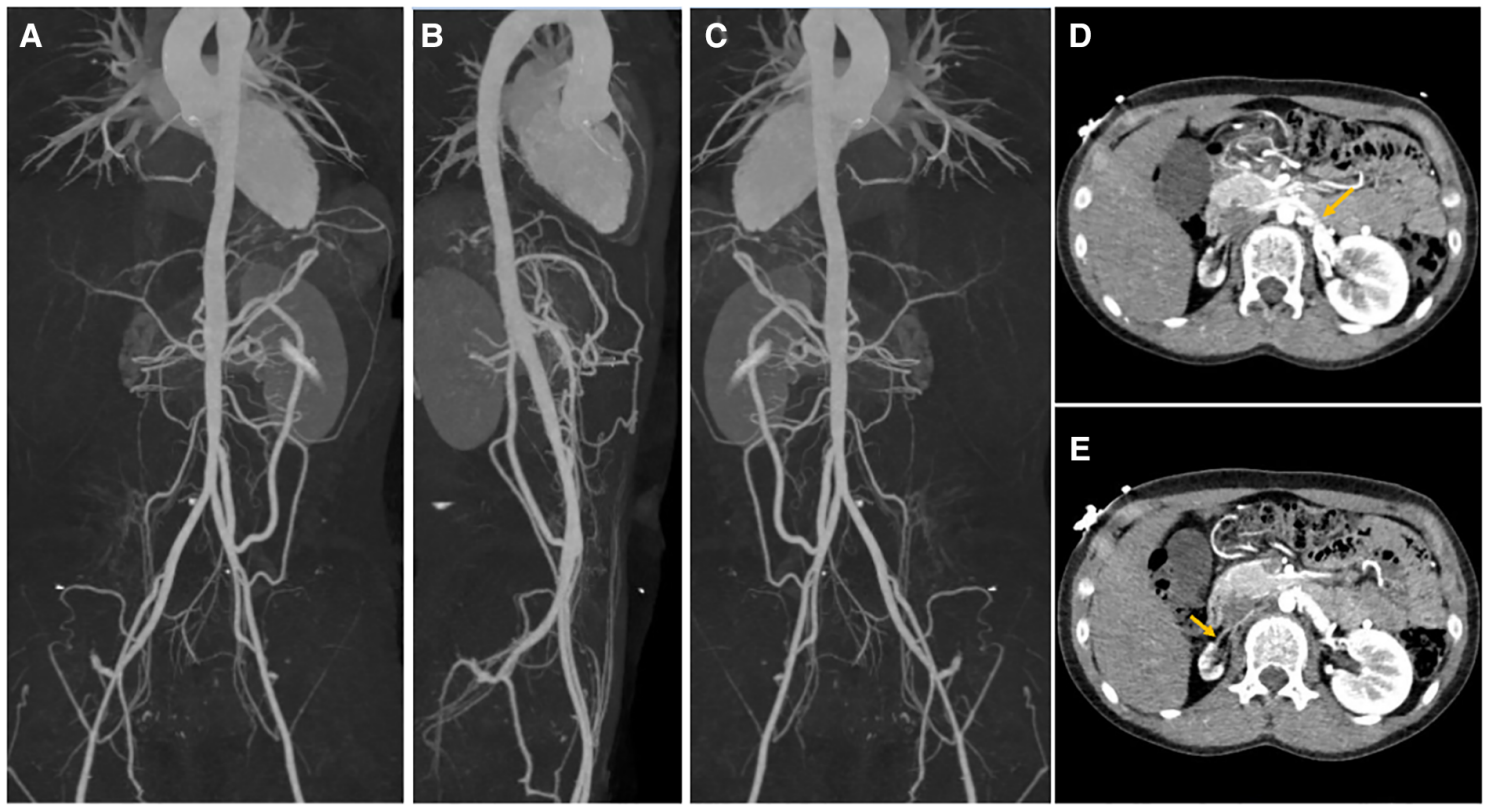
Stenosis of major and renal arteries in systematic CTA. The whole course of aorta was slender with multiple vascular thickening and stenoses. The diameter of the ascending aorta and was thoracic aorta about 21 and 12 mm, respectively. The wall of the aortic trunk and segments were thickened with calcification. Slim right renal artery was present with a diameter of about 1 mm and thickened renal arterial intima. (A) Anterior view, (B) lateral view, (C) posterior view of systematic CTA, (D) stenotic lesions of the left renal artery ostia, and (E) stenotic lesions of the right renal artery ostia. CTA, computed tomography angiography.
